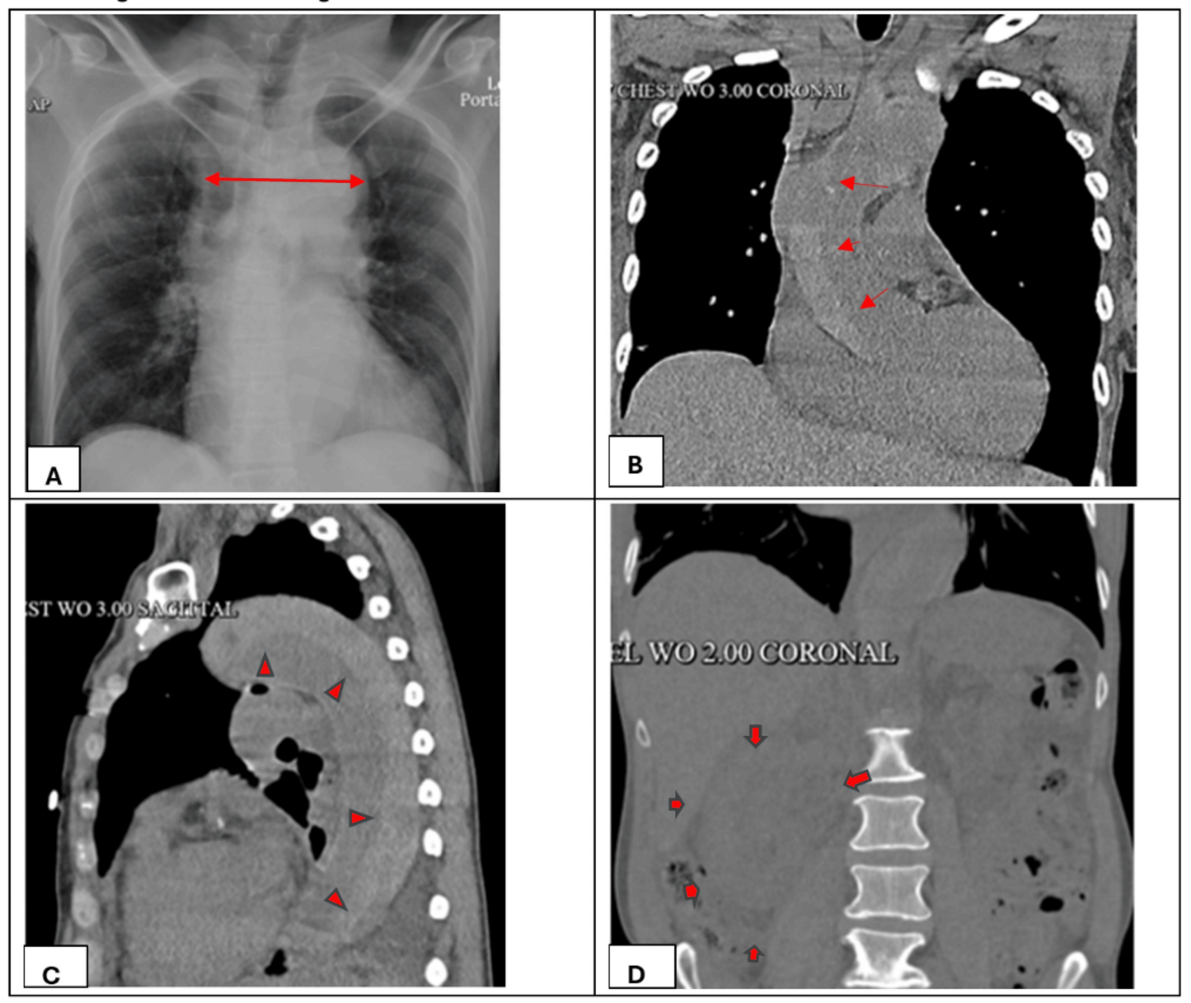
Images demonstrating extensive Stanford type A aortic dissection with signs of right kidney ischemia. (A) Chest X-ray showing mediastinal widening, initially reported as a prominent cardiomediastinal silhouette (red arrow). (B) Coronal plane CTA demonstrating a vertical intimal flap (red arrowheads) separating the true lumen from the false lumen, consistent with Stanford type A aortic dissection. (C) Sagittal plane CTA showing extensive aortic dissection extending from the aortic root to the abdominal aorta; red arrowheads indicate the dissection plane. (D) Coronal CT scan showing an enlarged right kidney with extensive perinephric fluid (red arrows). CTA: computed tomography angiography
